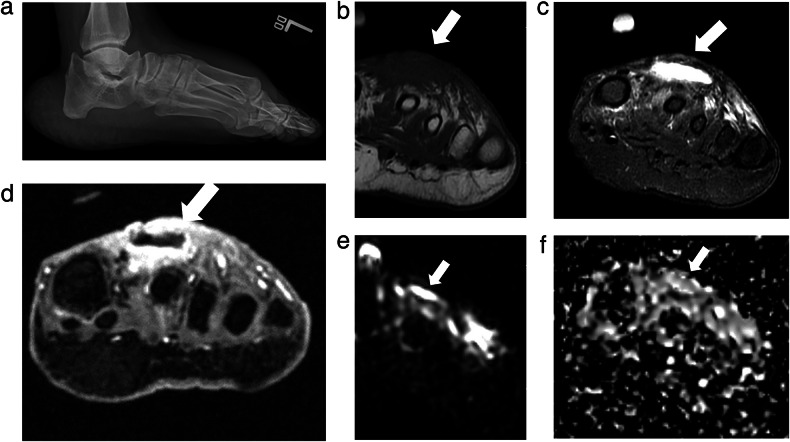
MSKI-RADS Class III. A 35-year-old man with foot infection. a Lateral X-ray of the affected left foot. b–d Axial T1W, fsT2W (water map Dixon), and 3D T1W axial post-contrast images of the left foot showing a peripherally enhancing collection (arrows), reflecting a subcutaneous abscess consistent with deeper soft tissue infection (class III). e, f Corresponding DWI and ADC map of the left foot show mild diffusion restriction of the area of cellulitis and abscess
